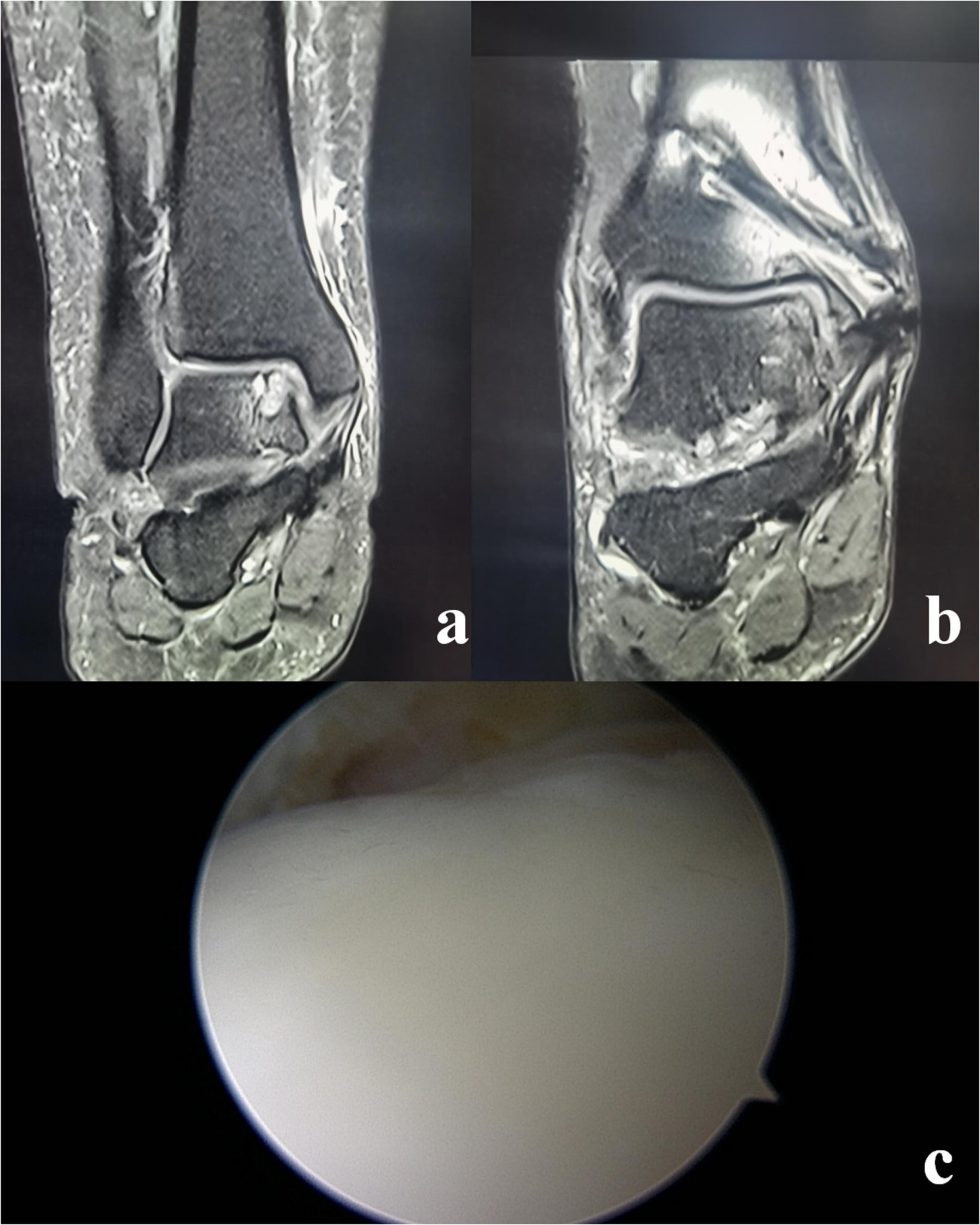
Typical and representative findings in a patient. (a) Magnetic resonance imaging (MRI) showed a large cartilage injury with a subchondral cyst on the medial zone of talus. (b) At the 2-year follow-up, MRI showed a healed graft with smooth cartilage surface. (c) Second-look arthroscopic examination showed nearly normal cartilage.
